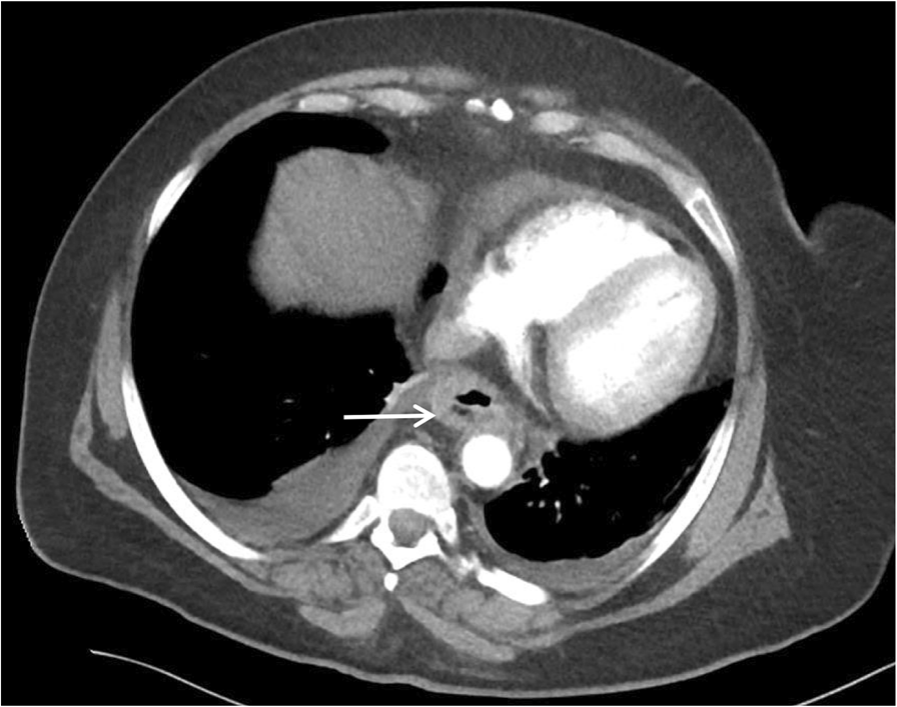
Computed tomography chest revealed extra-mural fat stranding and gas locules to the right lateral aspect of the gastro-oesophageal junction (arrow). Axial view
